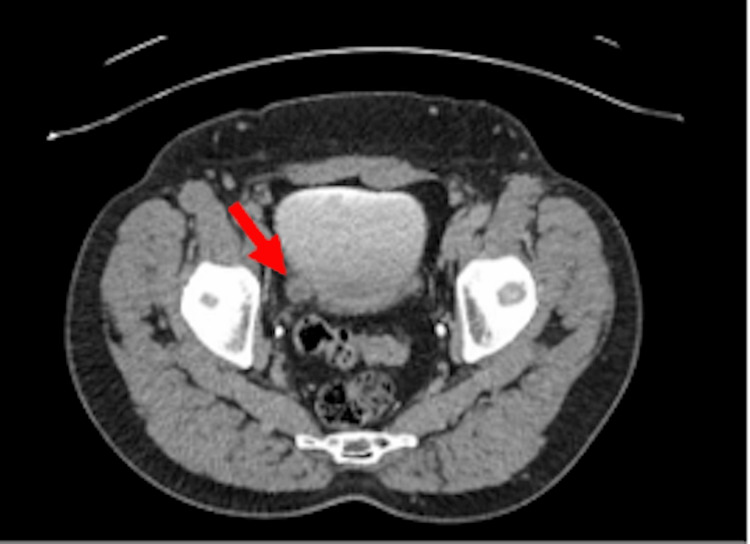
Computed tomography (CT) scan Post-contrast CT showing bladder diverticula (red arrow)
